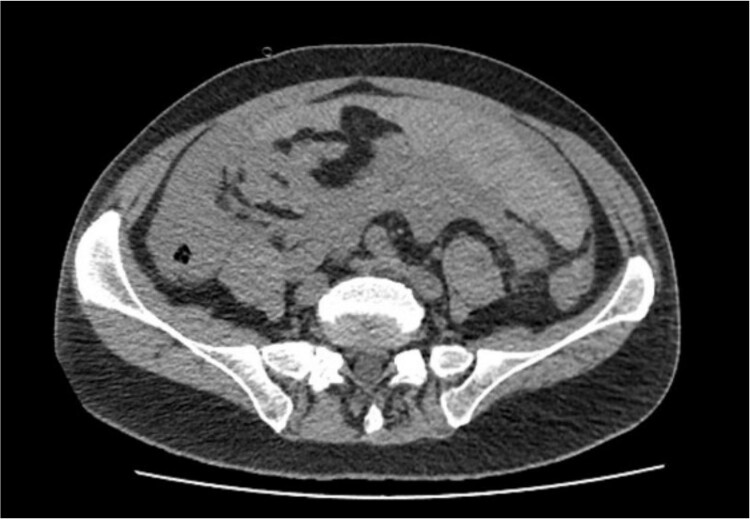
Computed tomography image on admission: moderate ascites with organized clot.
